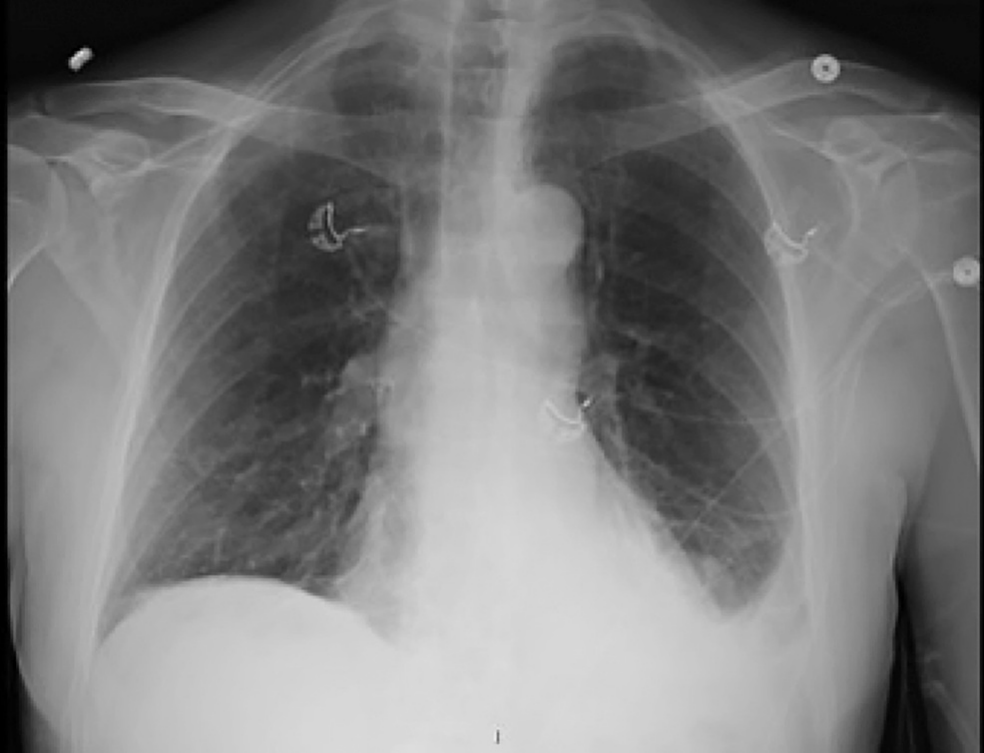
Chest x-ray with small left pleural effusion
